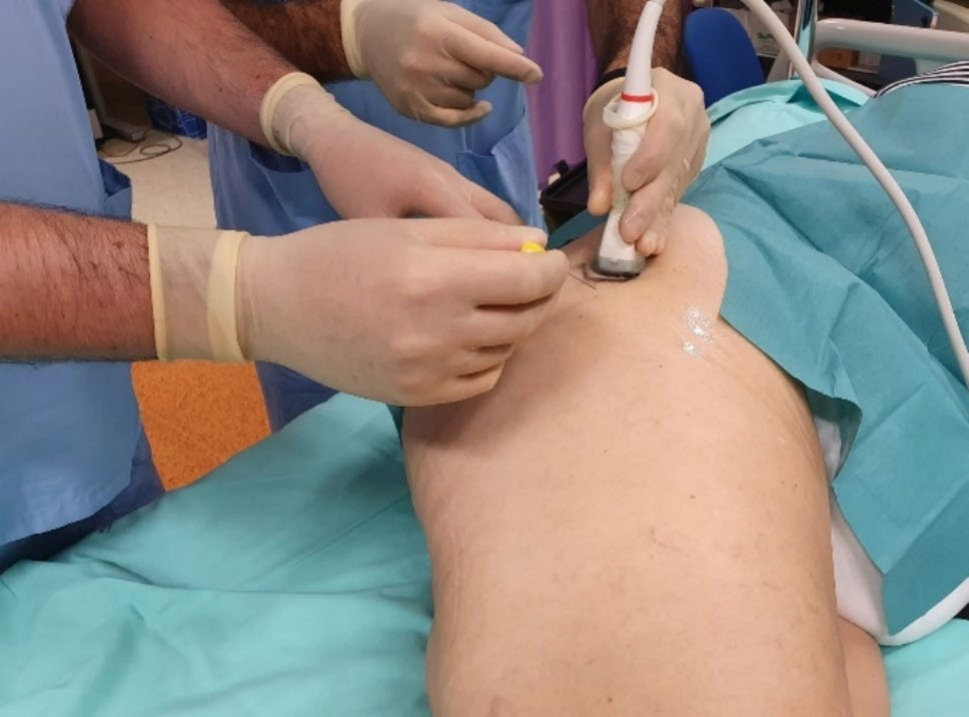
Ultrasound-guided intervention
